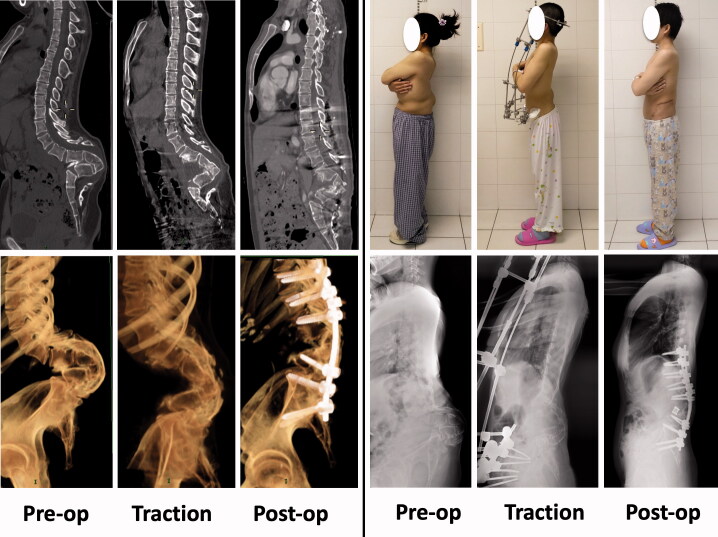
Case 1: Computed tomography (CT) revealed a congenital malformation in the lumbar region, and the lumbar spine appeared as if it was “completely folded up.” Treatment included HPT and anteroposterior fixation.
